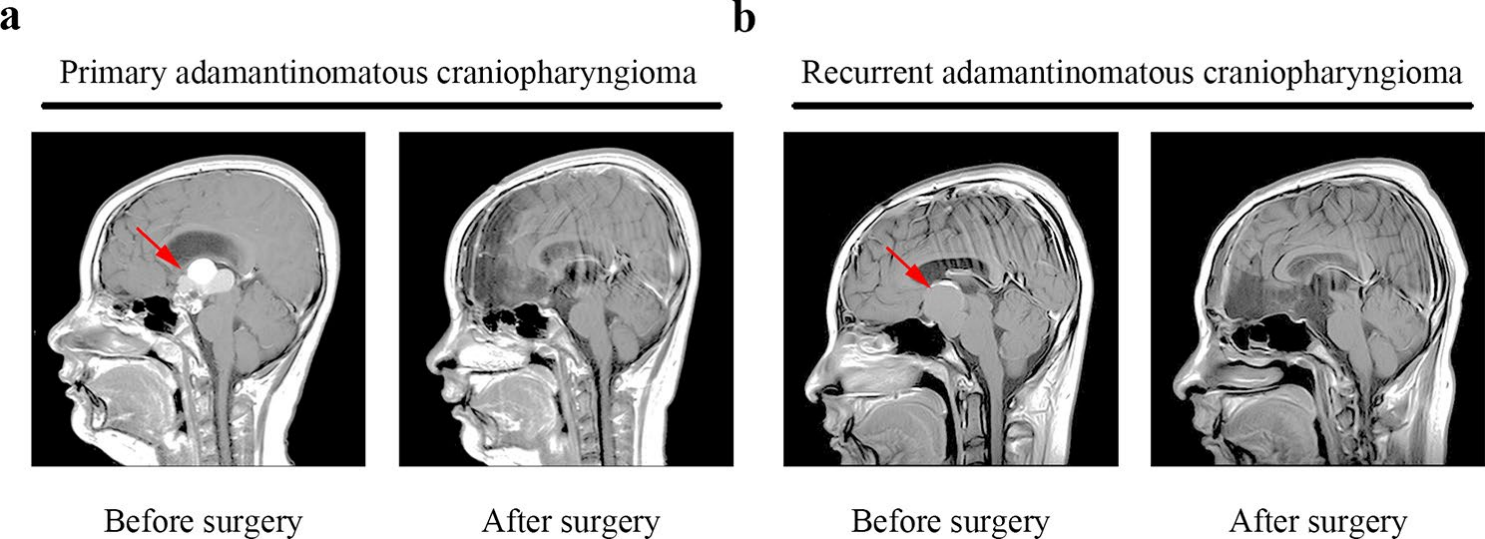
Representative magnetic resonance imaging of primary (a) and recurrent (b) adamantinomatous craniopharyngioma before and after surgery. Red arrows indicate tumour lesions
